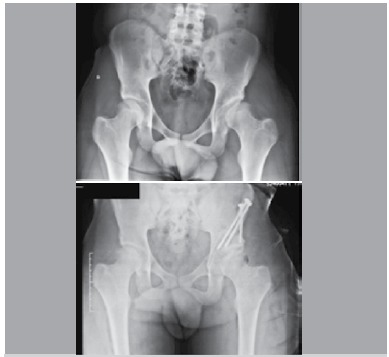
Case example: comparison of pre-operative and post-operative radiographs after a PAO done with a dual approach technique. The center-edge angle increased from 7 to 22 degrees.
